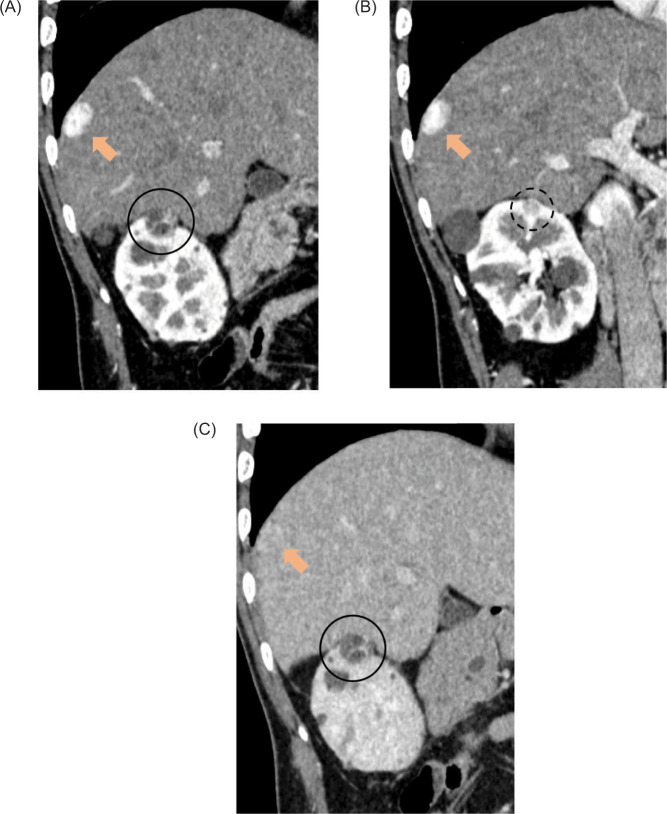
Dynamic computed tomography (CT) images (A and B: corticomedullary phase, C: delayed phase, oblique images). CT images revealed nodules with septal enhancement and cystic degeneration, 1.8 cm (circle) and 1.1 cm (dot circle) in diameter on the right renal upper pole. A nodule with early enhancement and delayed washout of 2.5 cm in diameter (arrow) bordering the liver surface was also detected.
